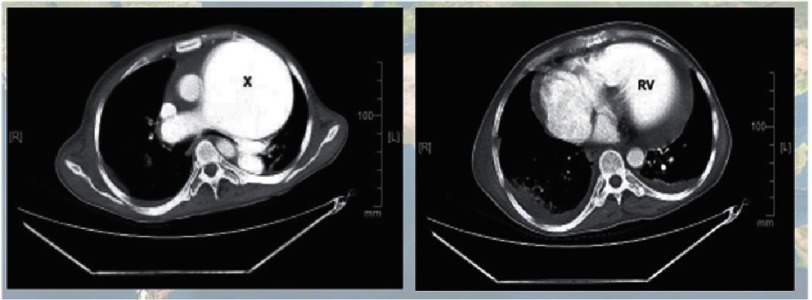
CT scan of patient with pulmonary hypertension due to schistosomiasis. The left panel shows severe pulmonary artery dilatation (X), and in the right panel shows the right ventricular dilatation and hypertrophy. (Images: courtesy of Dr T. Safwat).
